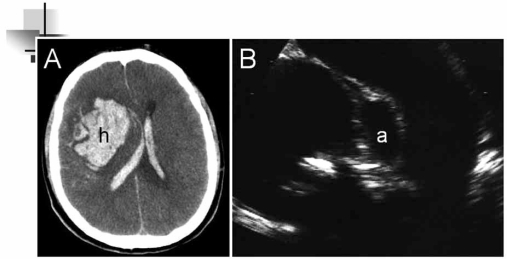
Right lobar hemorrhage (A) secondary to rupture of a mycotic aneurysm in the course of an infective bacterial endocarditis; transthoracic echocardiography (B) shows an abscess in the posterolateral aortic root (a) between the aortic valve leaflets and the mitral valve.
